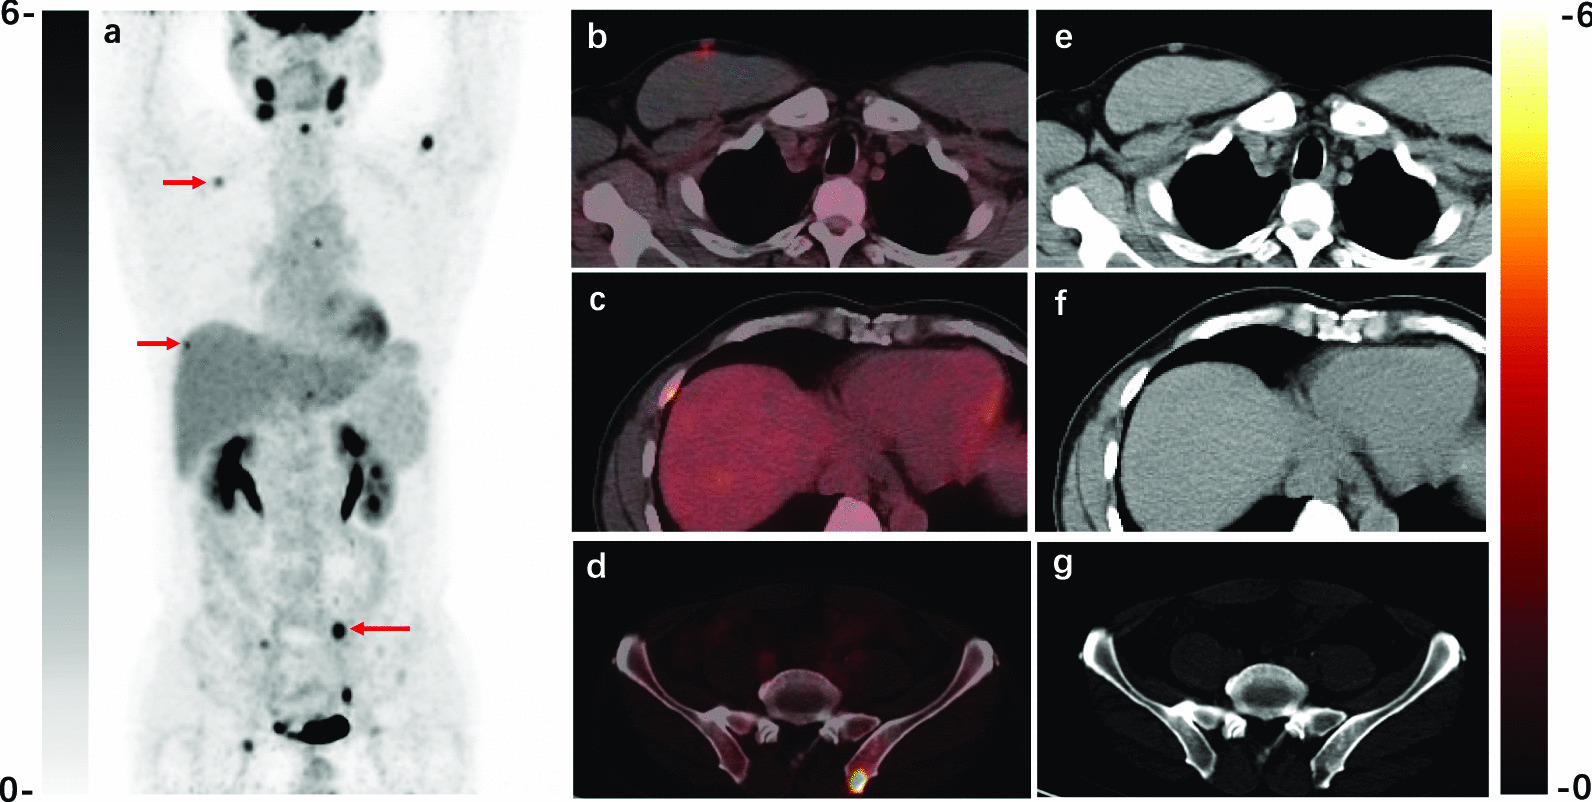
A typical RDD case with multisystem lesions revealed by ^18^F-FDG PET/CT. A 53-year-old man presenting with bilateral cervical lymph nodes enlargement was newly diagnosed with RDD by biopsy. The PET/CT images before treatment displayed hypermetabolic lymph nodes, subcutaneous nodules (b, e), and osteolytic lesions (c, d, f, g). He underwent chest CT before PET/CT, but these hypermetabolic nodules and bone lesions were neglected. The patient received lenalidomide and glucocorticoid due to multiple extranodal lesions
